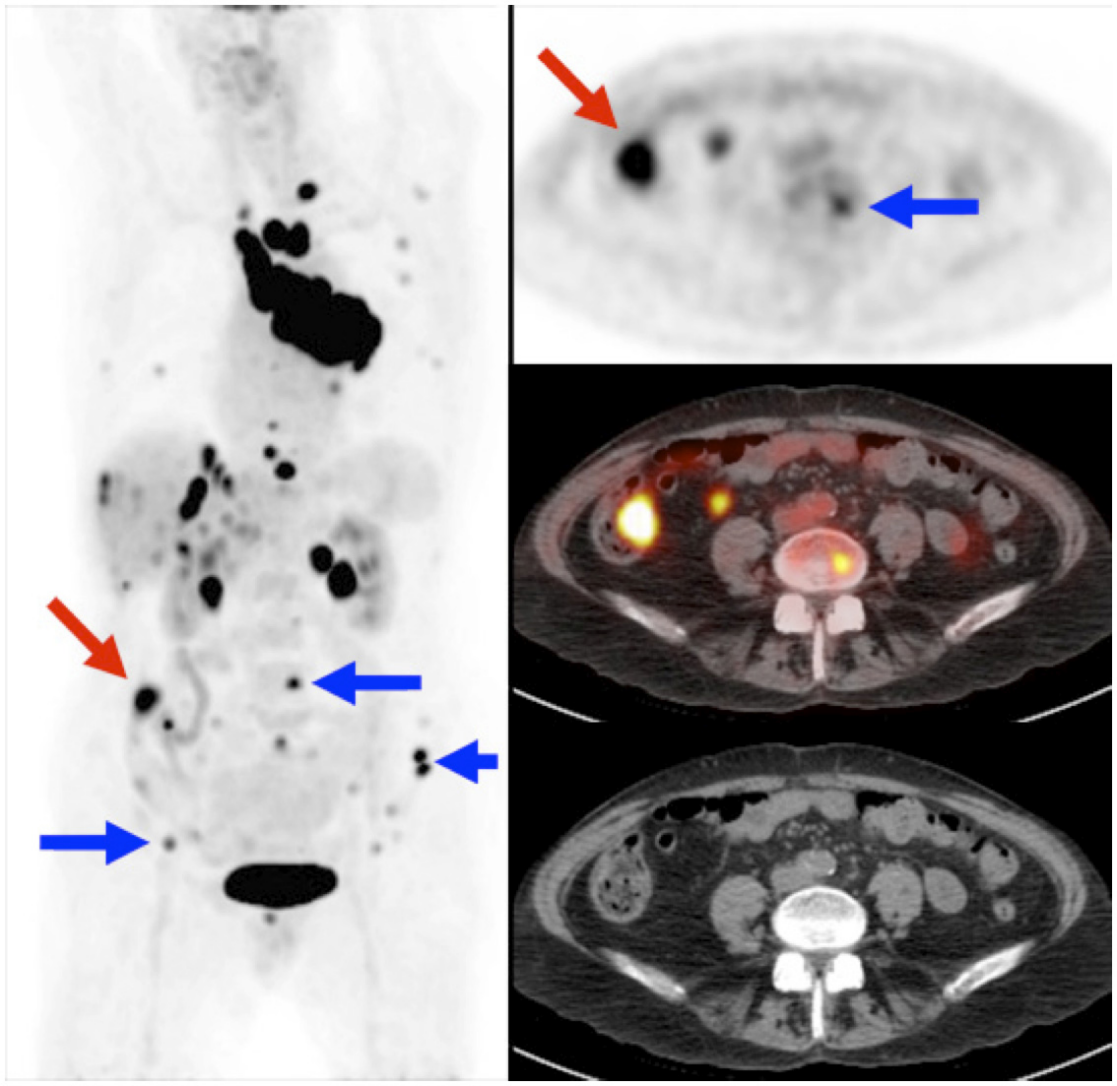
Example abdominal/pelvic area. True-positive but no-impact findings on PET/CT in the lower abdominal and pelvic area. Multiple skeletal metastases were detected (blue arrows), but these had no impact on staging as the thoracic field of view already showed multiple distant metastases (skeletal, adrenal glands, liver). An intense bowel hotspot (red arrow) is probably a colon carcinoma or large dysplastic polyp, but this was ignored as the prognosis of this patient was determined by the lung cancer. Imaging of the lower abdomen and pelvic area did not change the stage or therapy for this patient. Left: Coronal maximum intensity projection of PET. Right: Corresponding transverse slices of PET (top), PET/CT (middle), and CT (bottom).
